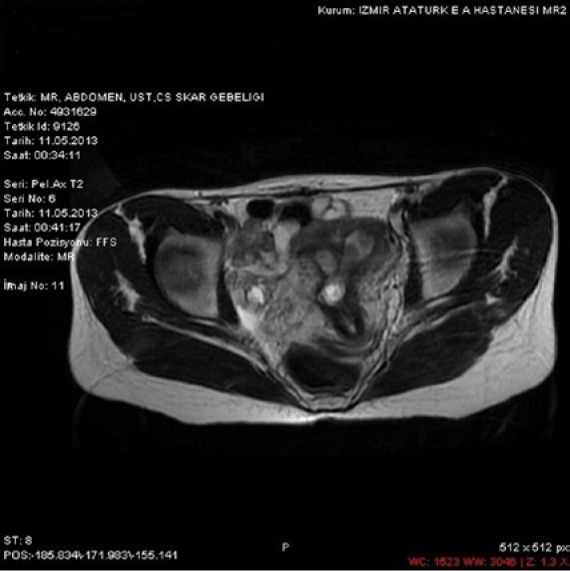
Magnetic resonance imaging examination of case 1.
